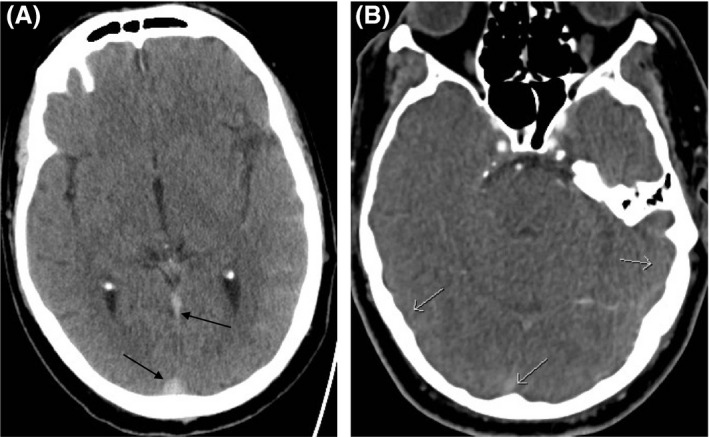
(A) Noncontrast‐enhanced CT shows subarachnoid hemorrhage in left temporal and parietal lobes and hyperdense thrombus in straight and superior sagittal venous sinuses (arrows). (B) CT venogram shows no opacification of superior sagittal sinus (arrow) or bilateral transverse sinuses (arrow), confirming venous sinus thrombosis.
